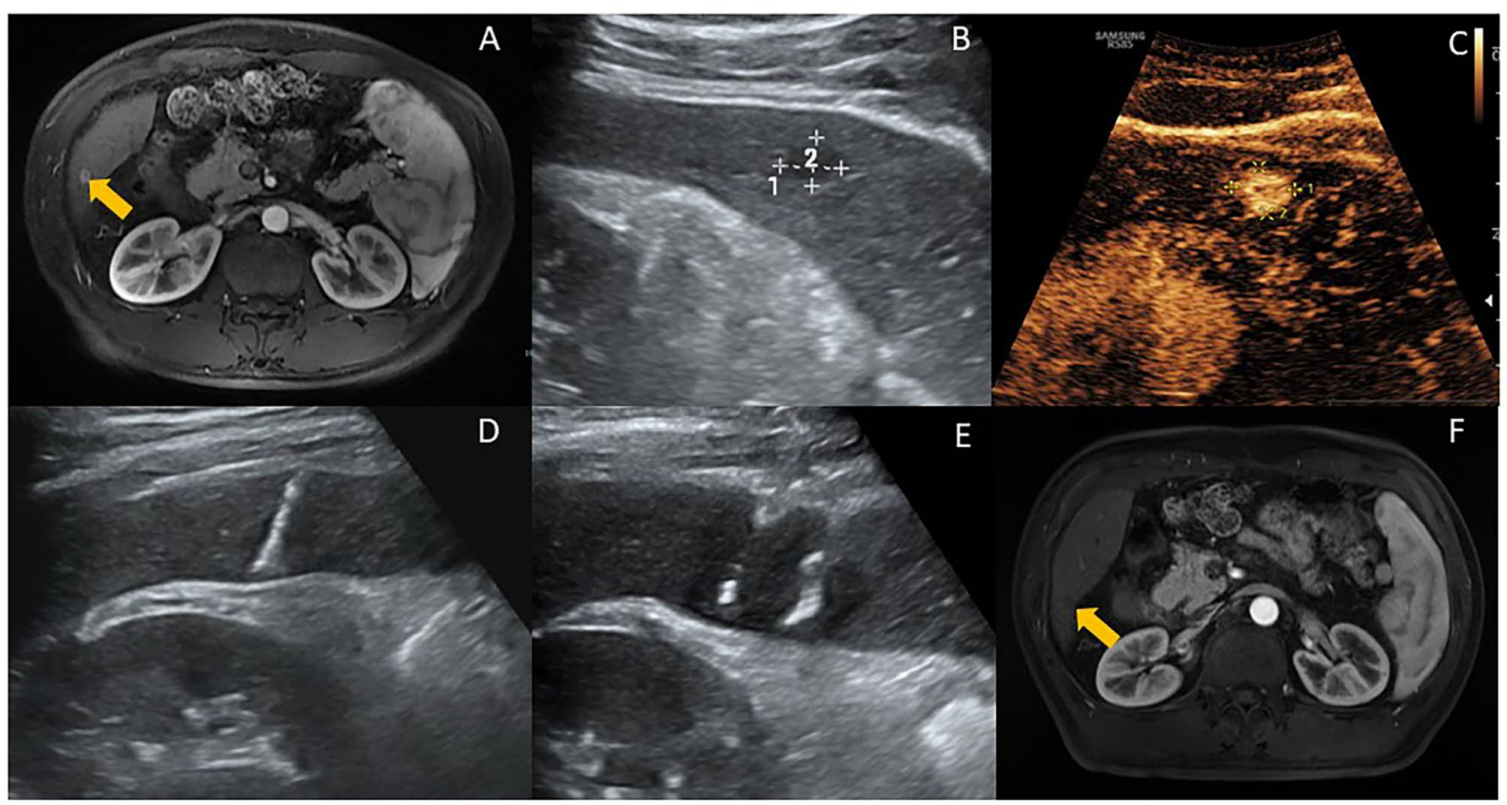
Ultrasound-guided IRE procedure for HCC. (A) Pre-ablation contrast-enhanced MRI revealed a hepatocellular carcinoma. (B&C) Ultrasound and Contrast-enhanced ultrasound showed a tumor located in S6 (arrow). (D&E) Ultrasound-guided IRE with a two-electrode configuration, and the head and tail end of the active tip of the electrodes can have punctuated enhancements (arrow). (F) The contrast-enhanced MRI results of one-month after the ablation revealed the tumor shrank obviously and no enhancement after ablation (arrow)
